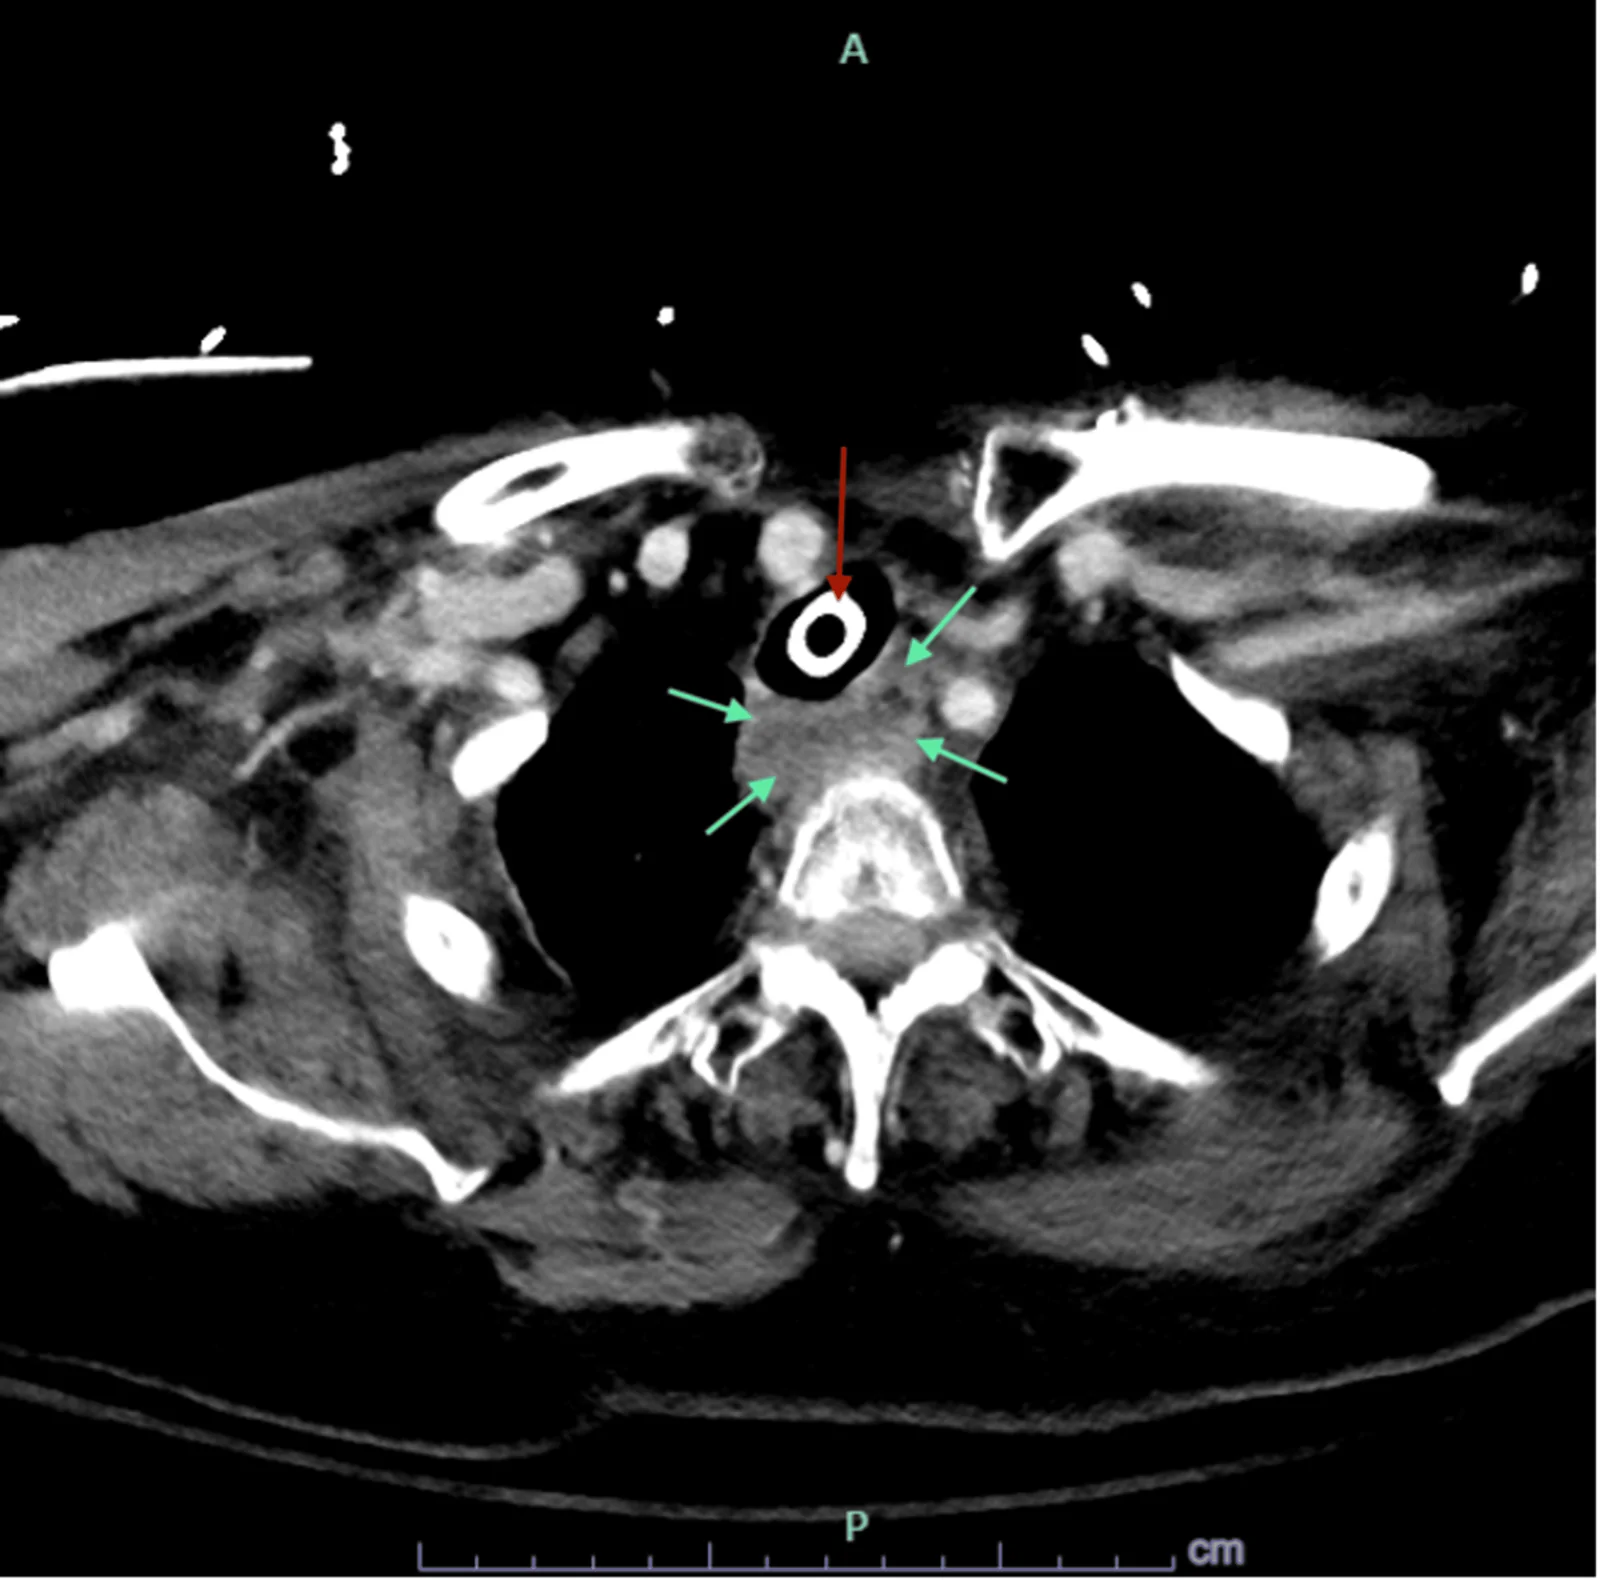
CT scan with contrast Circumferential esophageal edema post-esophageal perforation (green arrows). The patient’s tracheostomy tube (red arrow) can be seen in the trachea. A: anterior; P: posterior
